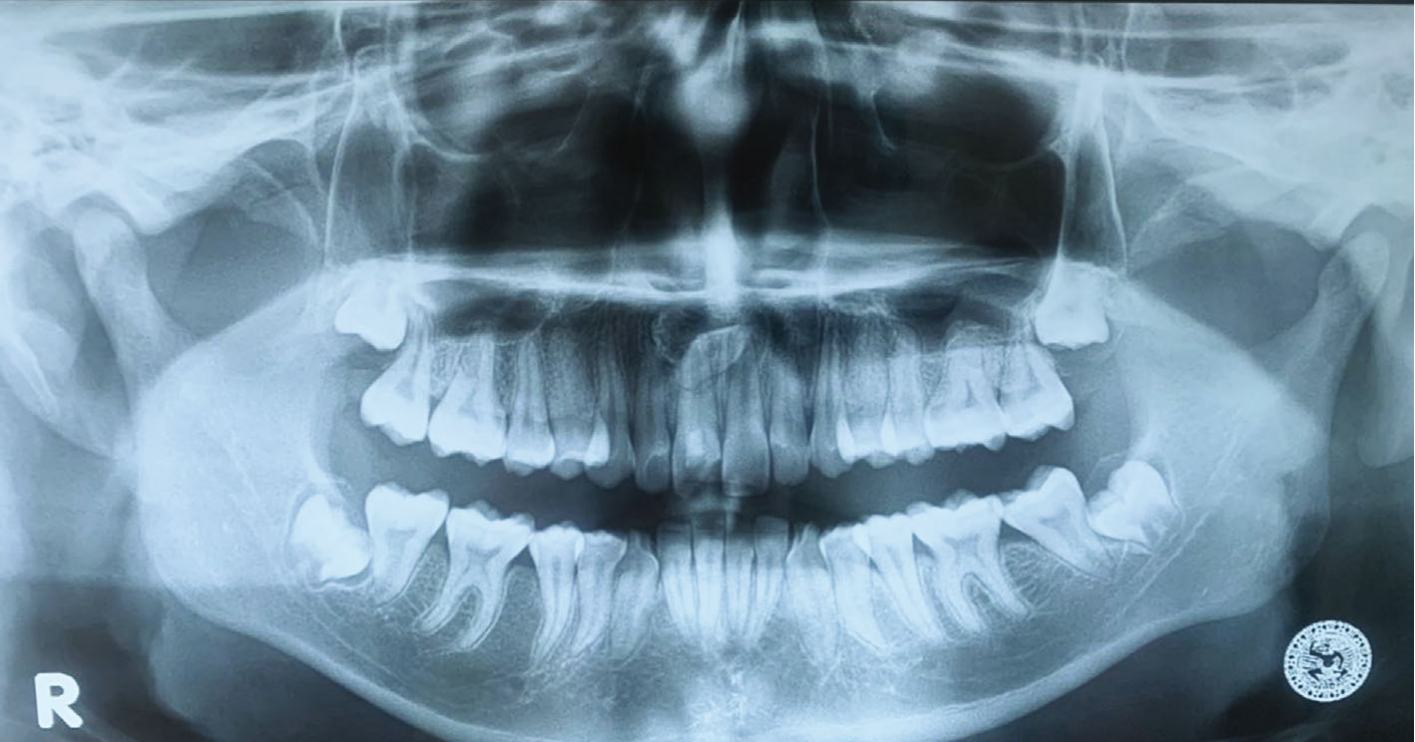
Panoramic radiographic view. Panoramic radiograph revealing a well-defined unilocular radiolucency around impacted mesiodens.
